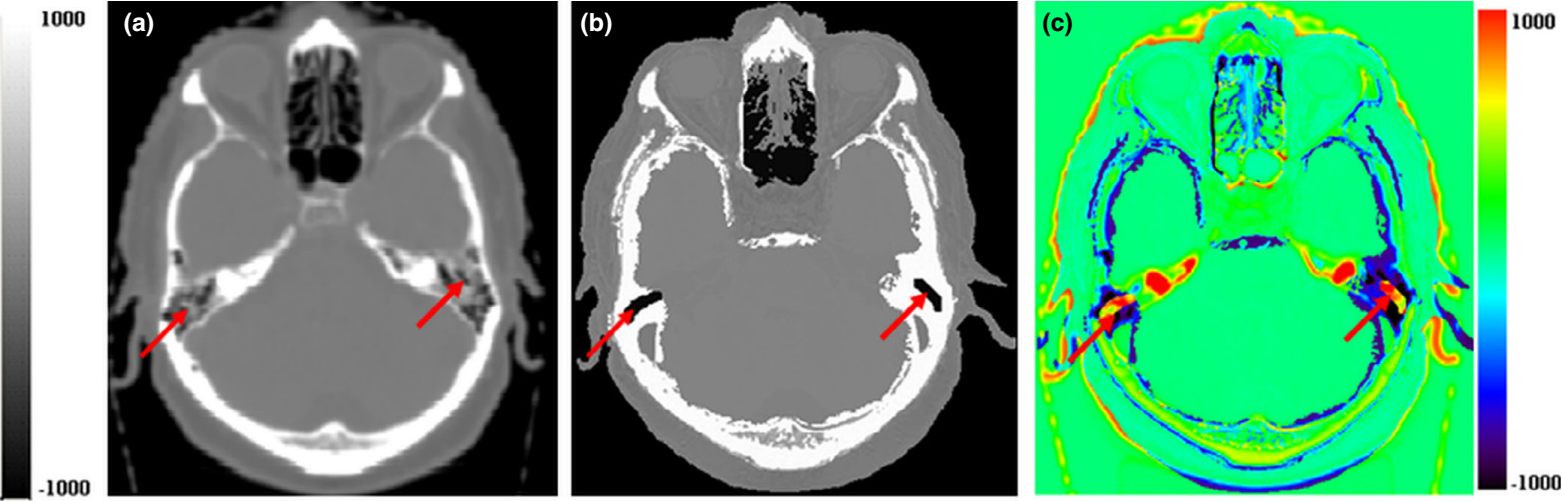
ED difference map between computed tomography (CT) and magnetic resonance. (a) CT slice, (b) synthetic‐CT slice, and (c) ED difference map between (a) and (b). Red arrows indicate mastoid sinuses
